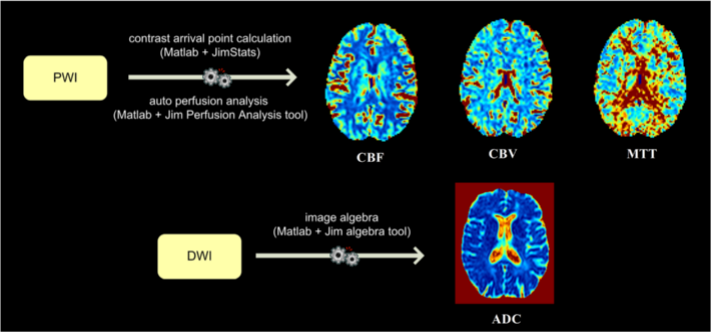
A descriptive example of generated apparent diffusion coefficient (ADC) and perfusion-weighted imaging (PWI) maps. CBF = Cerebral Blood Flow; CBV = Cerebral Blood Volume; MTT = Mean Transit Time; DWI = Diffusion Weighted Imaging
